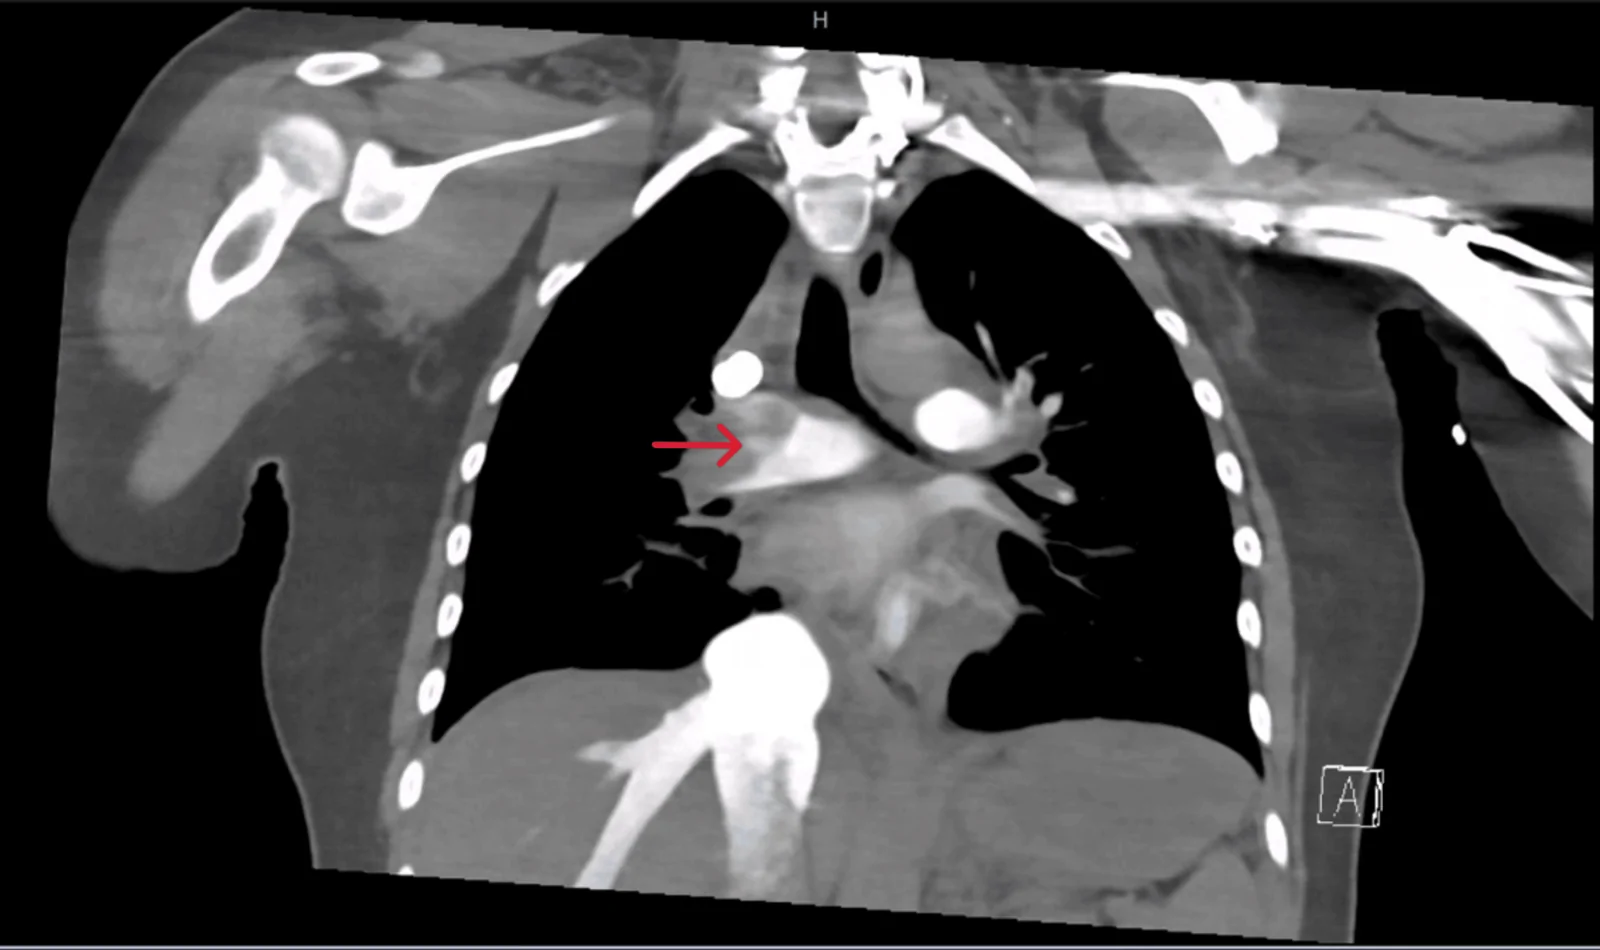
Computed tomography pulmonary angiography (CTPA) demonstrating extension of the embolus into the right main pulmonary artery (red arrow)
